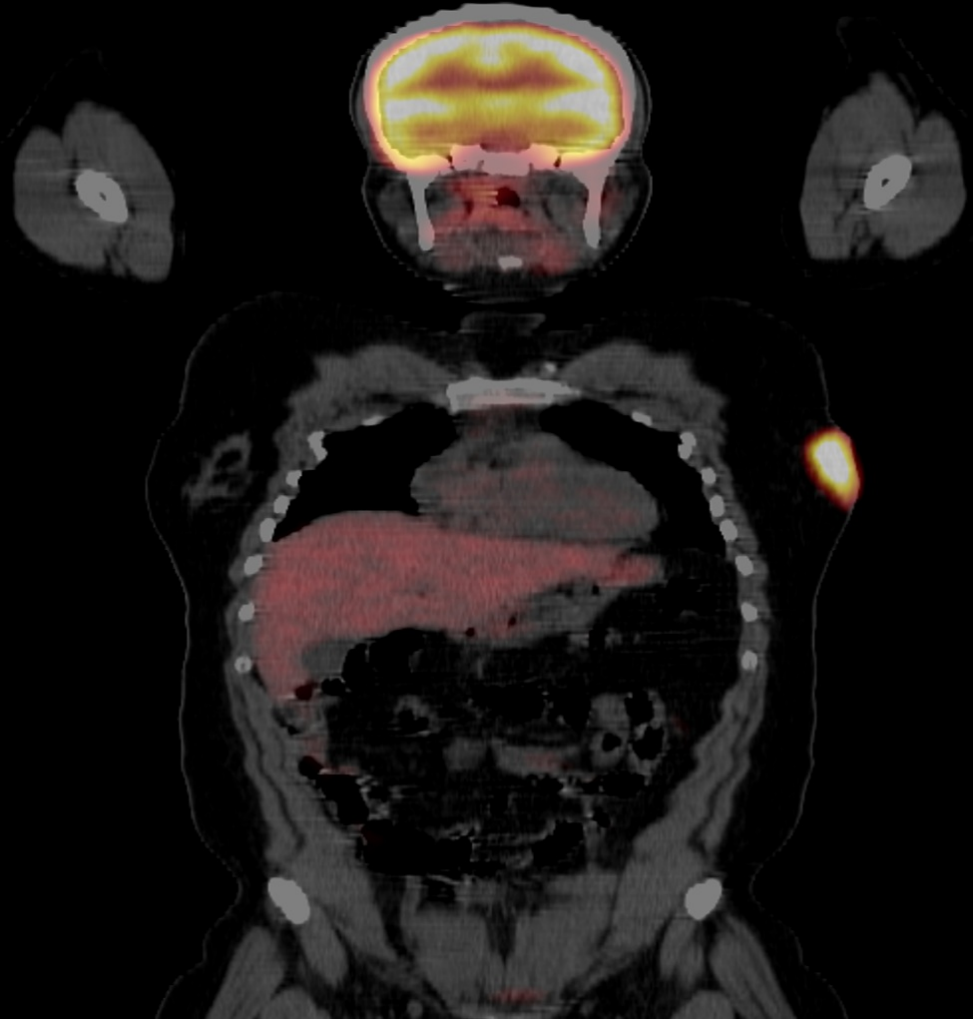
Baseline PET/CT scan showing lateral left breast uptake
